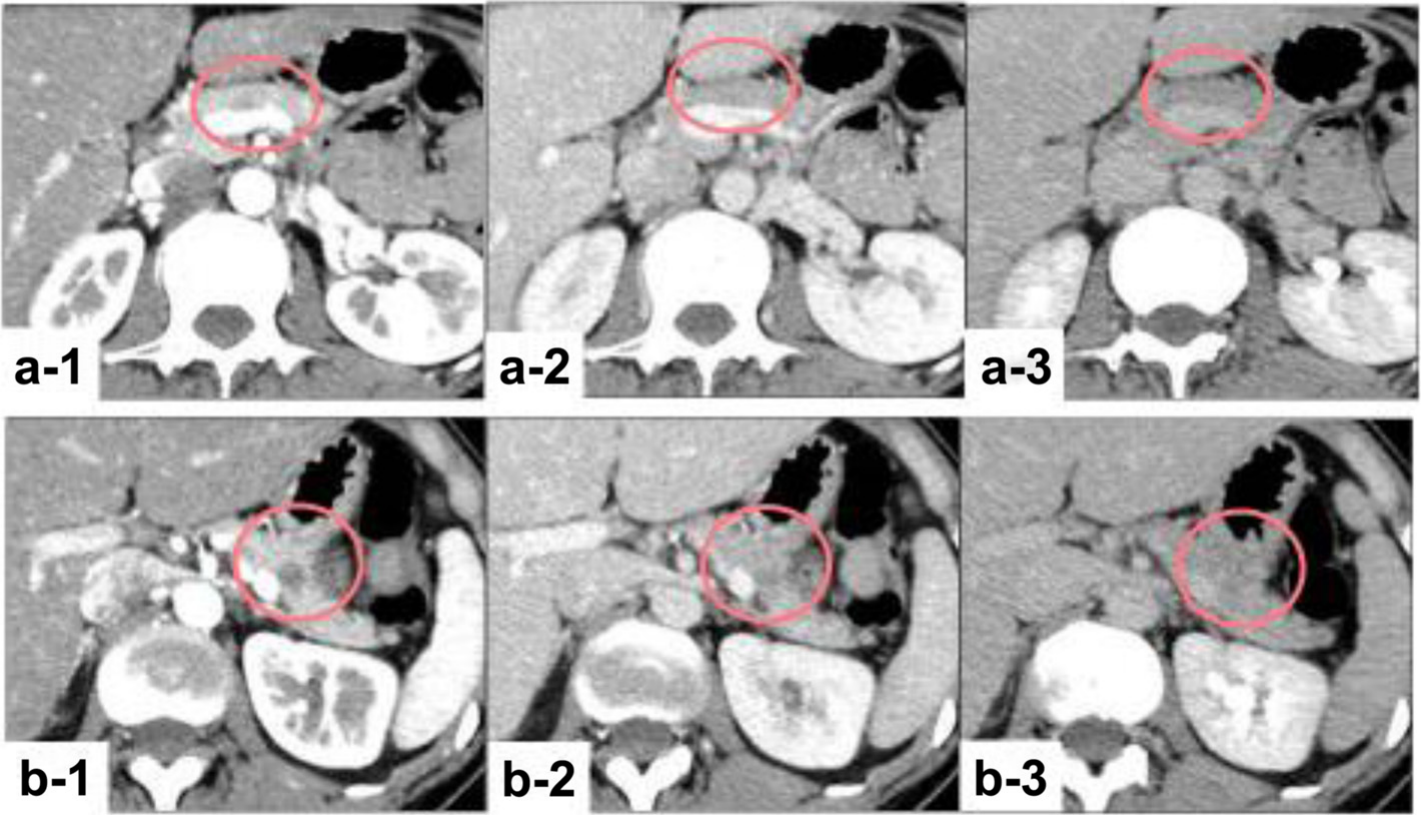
Contrast-enhanced computed tomography scan. (*a-1*) There was a 5-mm low-density mass in the pancreatic body. (*a-2*, *3*) This mass was gradually enhanced in the portal vein phase and the late phase. (*b-1*) There was also a 10-mm low-density mass in the pancreatic tail. (*b-2*, *3*) This mass had the same density and enhancement as the other mass
